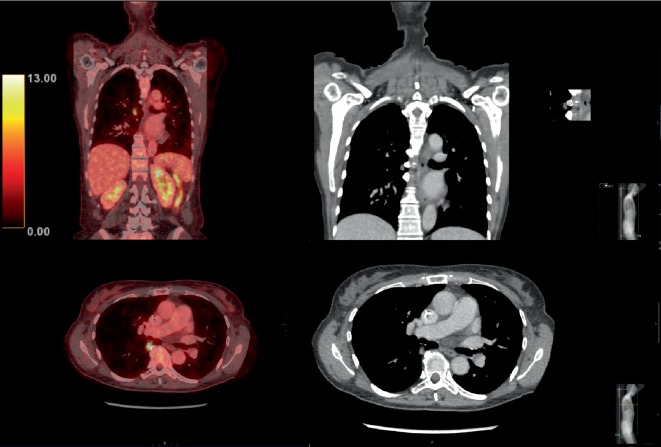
PET-CT evaluation of the response to first-line palliative chemotherapy showed disease progression with lung metastasis. PET-CT (left column) and CT (middle column) images. Top right inset: higher magnification image and measurement of the lesion. The SUVmax of the lesion was 9.7.
